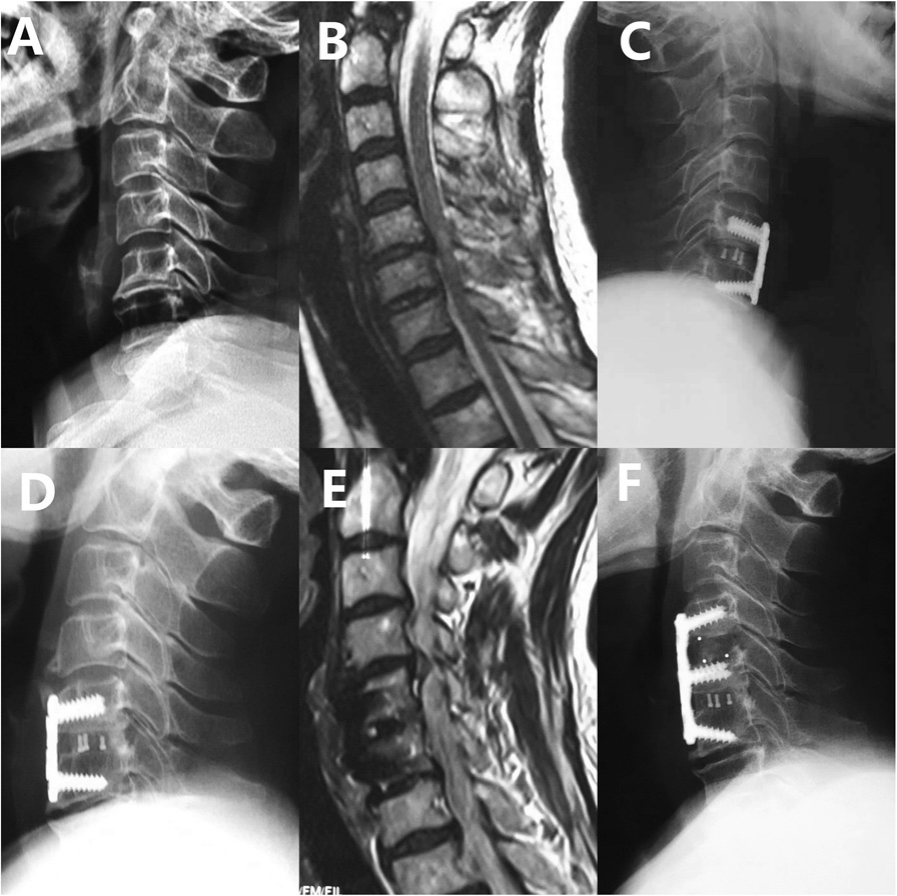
Non-DCS patient with recurrent myelopathic symptoms 8 years after anterior fusion of C5 to C6. a Preoperative radiograph shows non-DCS at any level. b Preoperative MRI of this 55-year-old female shows severe compressions of the spinal cord at C5-C6 level as well as high signal intensity in the spinal cord. c Radiograph after initial surgery shows ACDF at C5-C6. d Radiograph 8 years after initial surgery shows osseous fusion of C5 to C6 and development of ASD at C4-C5. e MRI at 8-year follow-up shows development of ASD and spinal cord compression at C4-C5. f Radiograph after the second surgery shows ACDF at C4-5. ACDF, anterior cervical decompression and fusion; ASD, adjacent-segment degeneration; DCS, developmental canal stenosis, MRI, magnetic resonance imaging
